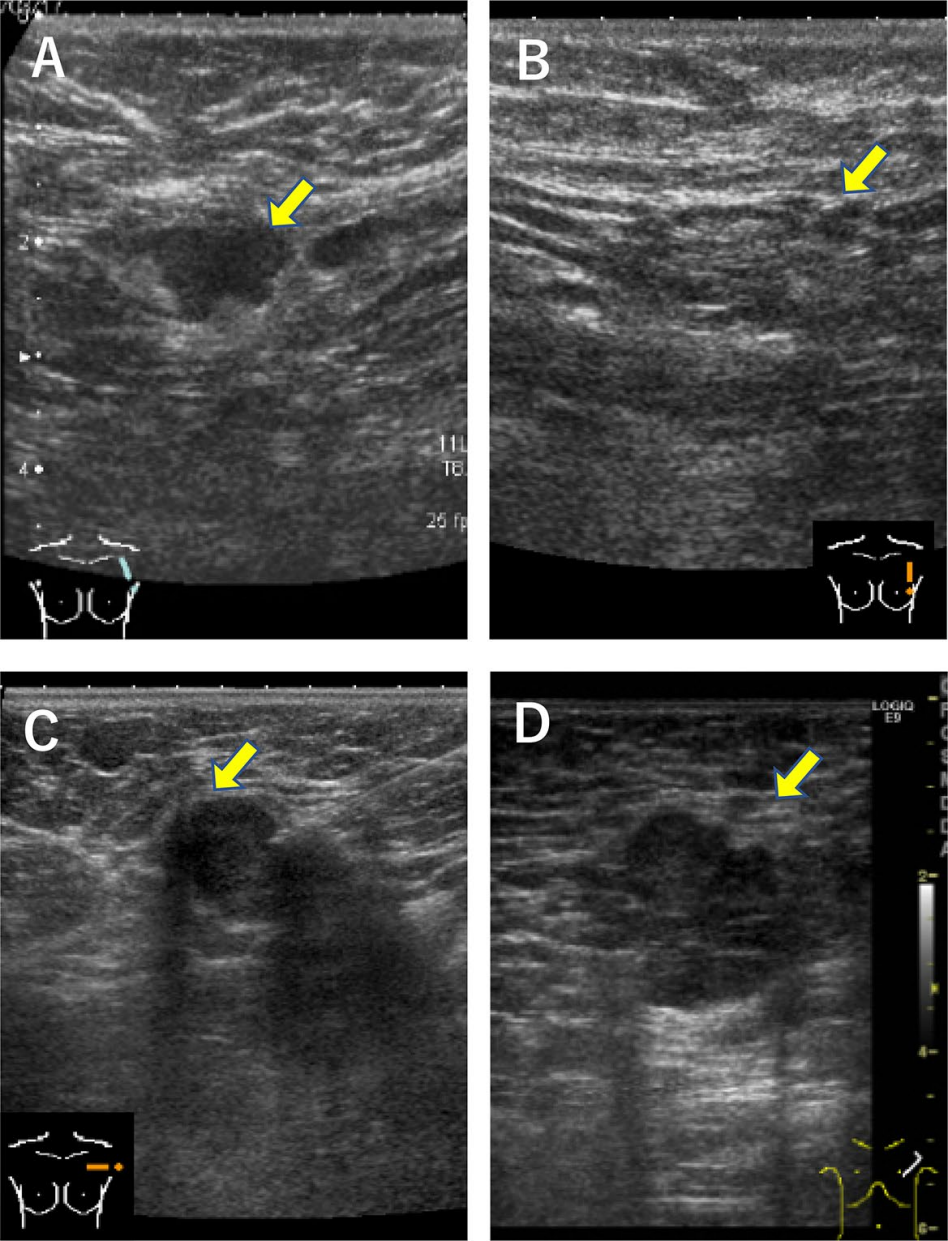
Representative ultrasound images. Patient 1. Before NAC, oval shaped lymph node was detected, which was cytologically confirmed as metastatic node (A). After NAC, lymph node shrunk, which was pathologically confirmed as negative node (B). Patient 2. Before NAC, ultrasound image of an axillary lymph node on fine needle aspiration showed irregular shaped lymph node, which was cytologically confirmed as metastatic node (C). After NAC, irregular shaped lymph node remained, which was pathologically confirmed as positive node (D).
